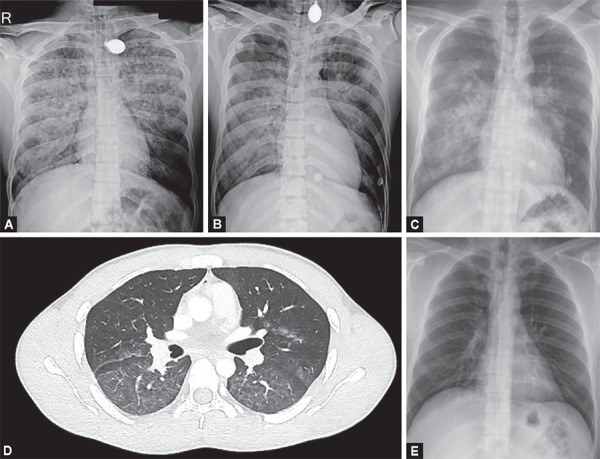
(A) Initial chest X-ray showing pulmonary edema; (B) Chest X-ray after 24 hours; (C) Chest X-ray after extubation when patient is on NIV; (D) HRCT showing diffuse GGO; (E) Chest X-ray after 7 days showing complete resolution
